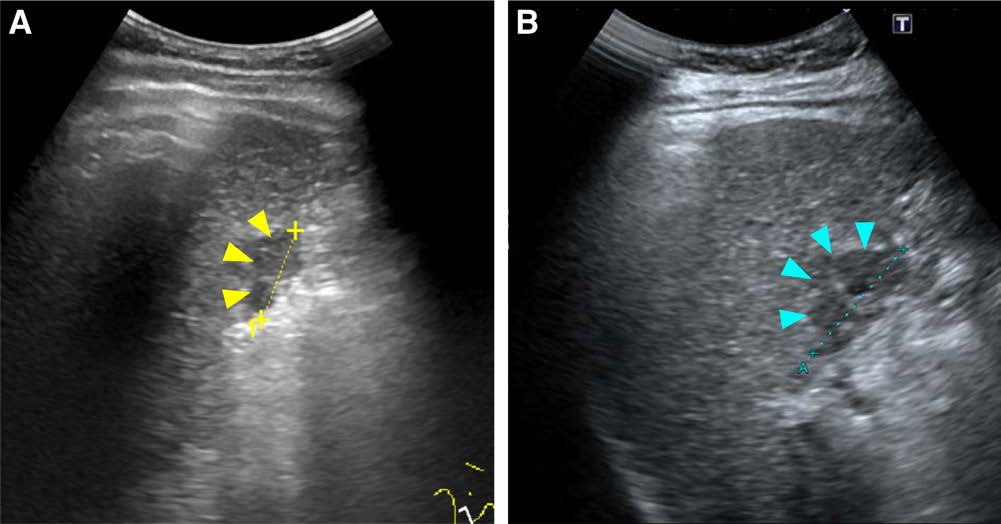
Ultrasound follow-up of the hepatic tumor. Abdominal ultrasonography showed an enlarging hypoechoic mass near segment 5 of the liver, measuring 24 mm (A, yellow arrowhead) and 35 mm (B, blue arrowhead) within three months.
